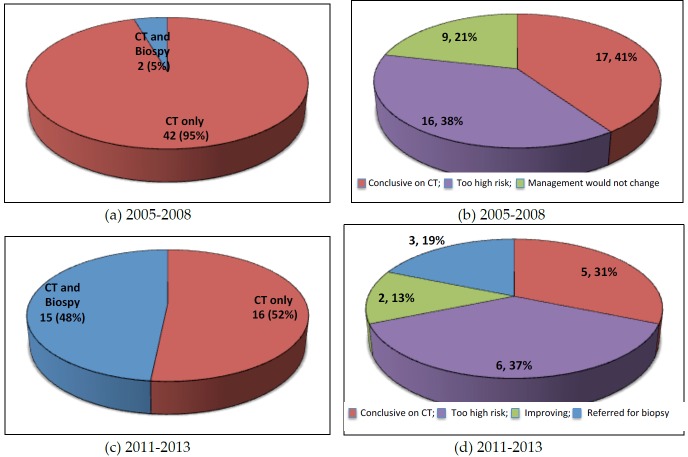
The multidisciplinary discussion and diagnosis of interstitial lung disease of unknown classification: (a) The modalities used to make the diagnosis in 2005–2008; (b) The reasons why biopsy was not performed when diagnosis was made by CT imaging; (c) The modalities used to make the diagnosis in 2011–2013; (d) The reasons why biopsy was not performed if diagnosis was made by CT imaging alone.
